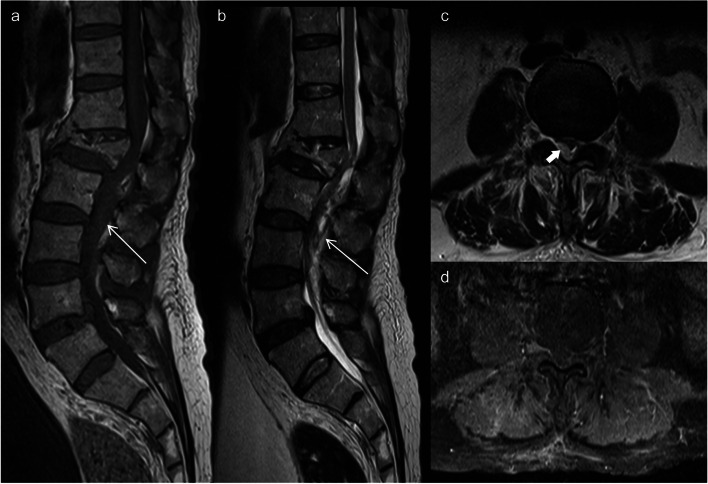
Intrathecal methotrexate (MTX) administration complicated by epidural hematoma. A 66-year-old man was on an intrathecal MTX regimen for treatment of non-Hodgkin lymphoma, presented with severe back pain that radiates to the left leg after the first week of intrathecal MTX administration. Sagittal T1- (a) and T2-weighted (b) MRI lumbar spine images demonstrate a posterior epidural collection of iso to slightly high T1 and mixed heterogeneous T2 signal intensities, compressing the thecal sac with crowding of cauda equina (arrows). Axial T2WI (c) shows corresponding high T2 intensity epidural collection compressing the right posterolateral aspect of the thecal sac (thick arrow). Axial T1W post-contrast image reveals no abnormal enhancement. The findings are consistent with an epidural hematoma. Besides, there is an old compression fracture of the L1 vertebral body which has been stable since the prior study (not shown). Patient underwent an urgent laminectomy and evacuation of the epidural hematoma with subsequent improvement of his symptoms
